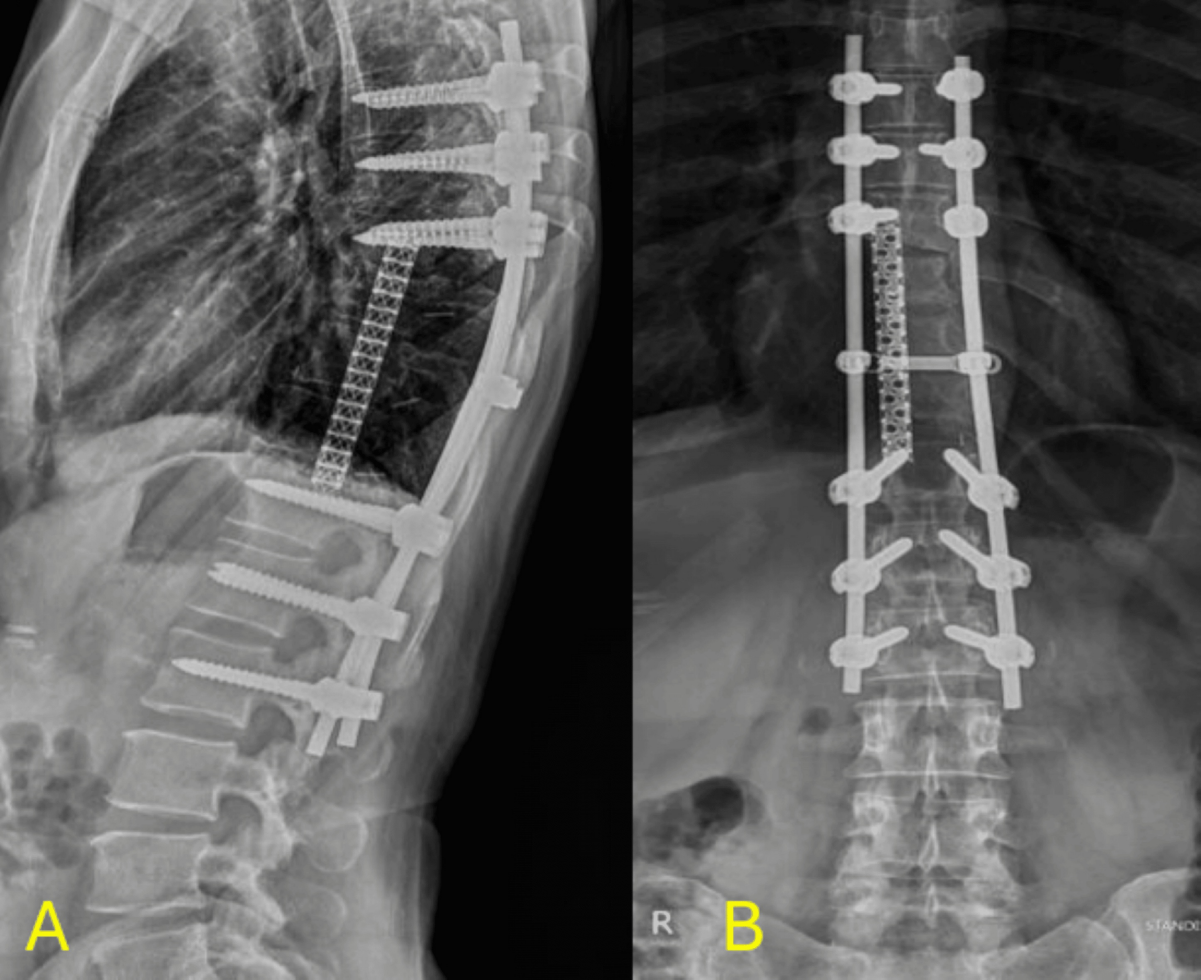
X-rays at the one-year follow-up X-ray at the one-year follow-up showing the integrity of the mesh cage's anterior and posterior instrumentation 5A: Sagittal view X-ray of the mesh and spinal instrumentation at the one-year follow-up 5B: Coronal view X-ray of the mesh and spinal instrumentation at the one-year follow-up
